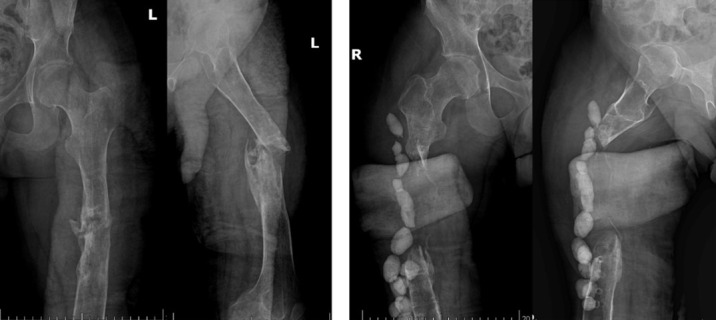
Preoperative radiographs of both femurs showing bilateral chronic non-union with segmental bone defects, more pronounced on the right side, and antibiotic beads in situ in the right femur: left femur AP and lateral views (left) and right femur AP and lateral views (right).
